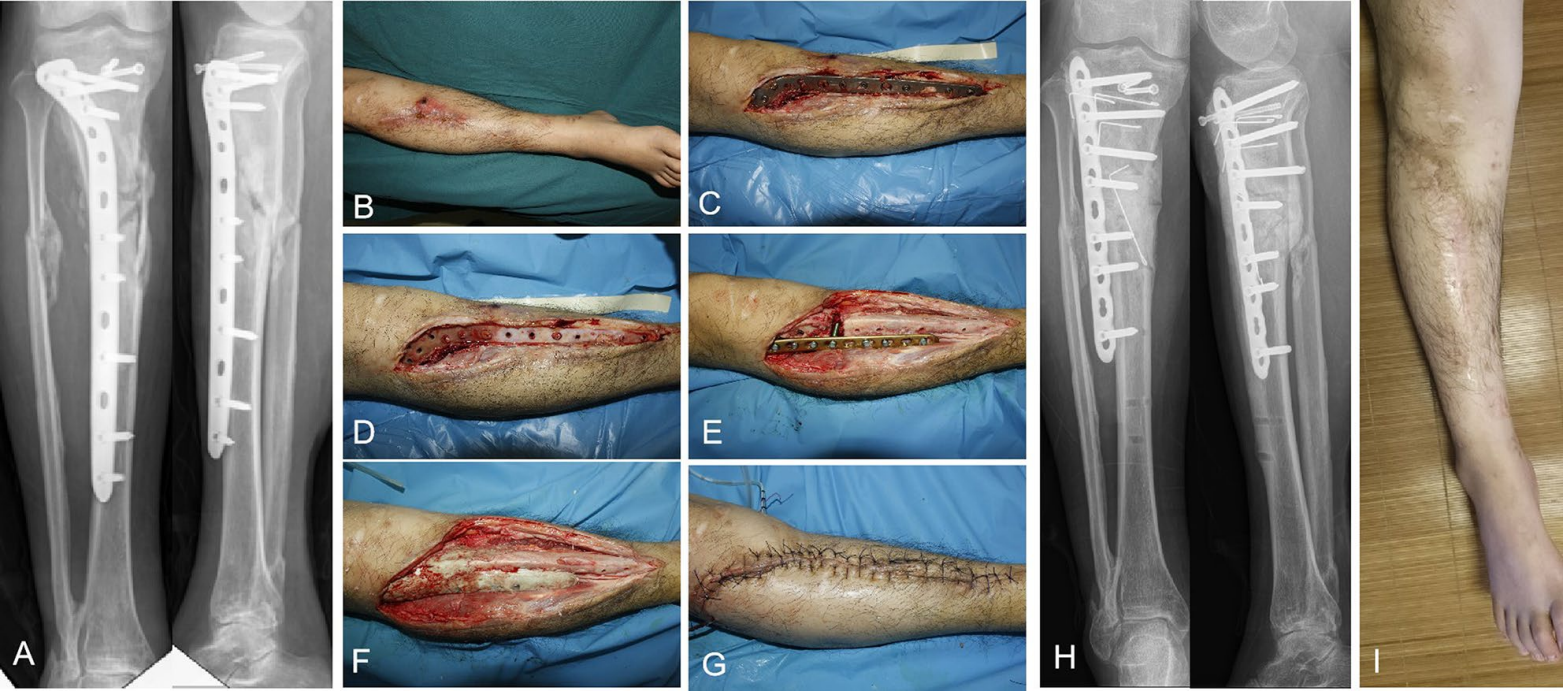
Typical case, Male, 25Y, repeatedly ulceration and pus of the right leg for 1 year, (A) X-rays showed bone destruction, (B) photo before operation, (C) and (D) infected tissue showed after removal of the internal fixation, E, bone defect was stabilized with LCP after debridement, (F) antibiotic cement filled the defect and wrapped the plate, (G) sutures the incision, (H) postoperative X-ray, (I) the incision healed at 6 months follow up.
